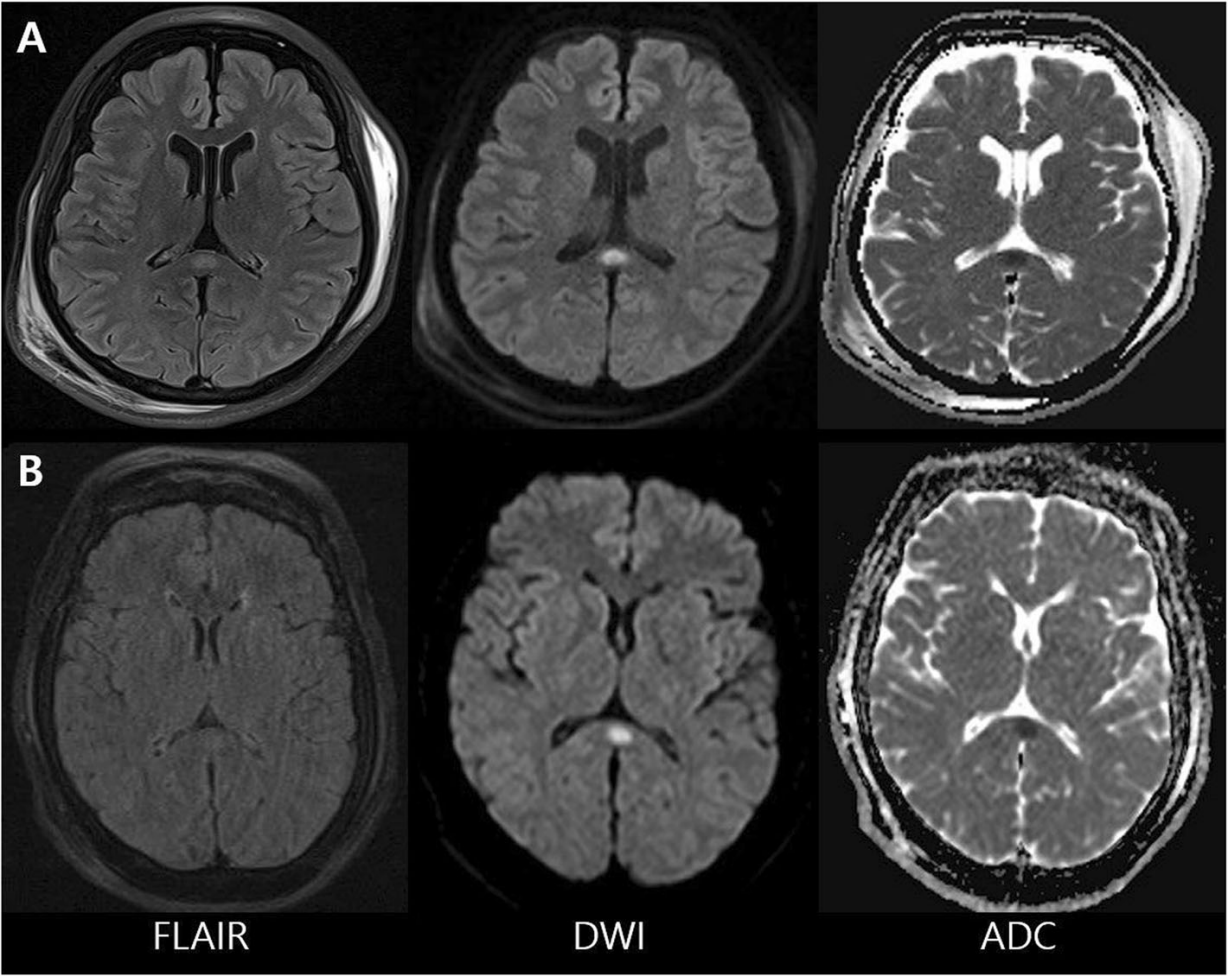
Brain magnetic resonance imaging of the two patients (a, Case 1; b, Case 2). Diffusion weighted imaging in both demonstrated a high signal intensity lesion in the mid portion of the splenial corpus callosum and showed low signal intensity on the apparent diffusion coefficient map, suggestive of cytotoxic edema
